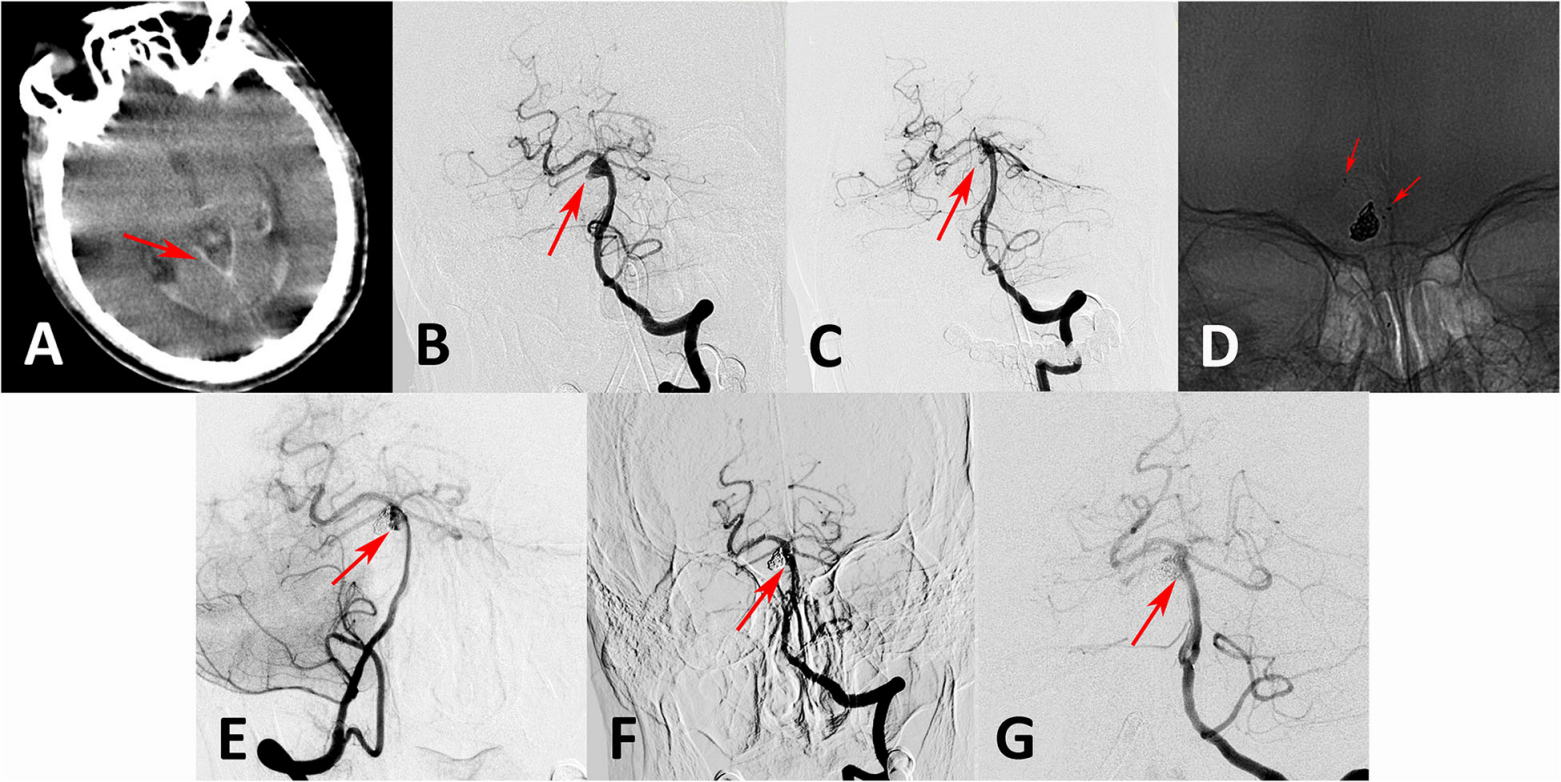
A ruptured basilar trunk aneurysm with a daughter sac was treated with LVIS-assisted coiling, and computed tomography confirmed subarachnoid hemorrhage (a arrow). Compared with the anteroposterior position in the preoperative angiographic images (b arrow), the aneurysm was completely occluded after treatment in the immediately post-procedure angiograph (c arrow). The LVIS stent was placed across the neck of the aneurysm followed by embolization with coils (d). At the 6-month follow-up angiography, the aneurysm had recanalized (e arrow). Repeat coiling was performed, and the residual aneurysm neck was occluded completely (f arrow). At the 7-month follow-up angiography, the aneurysm was stable and completely occluded (g arrow)
